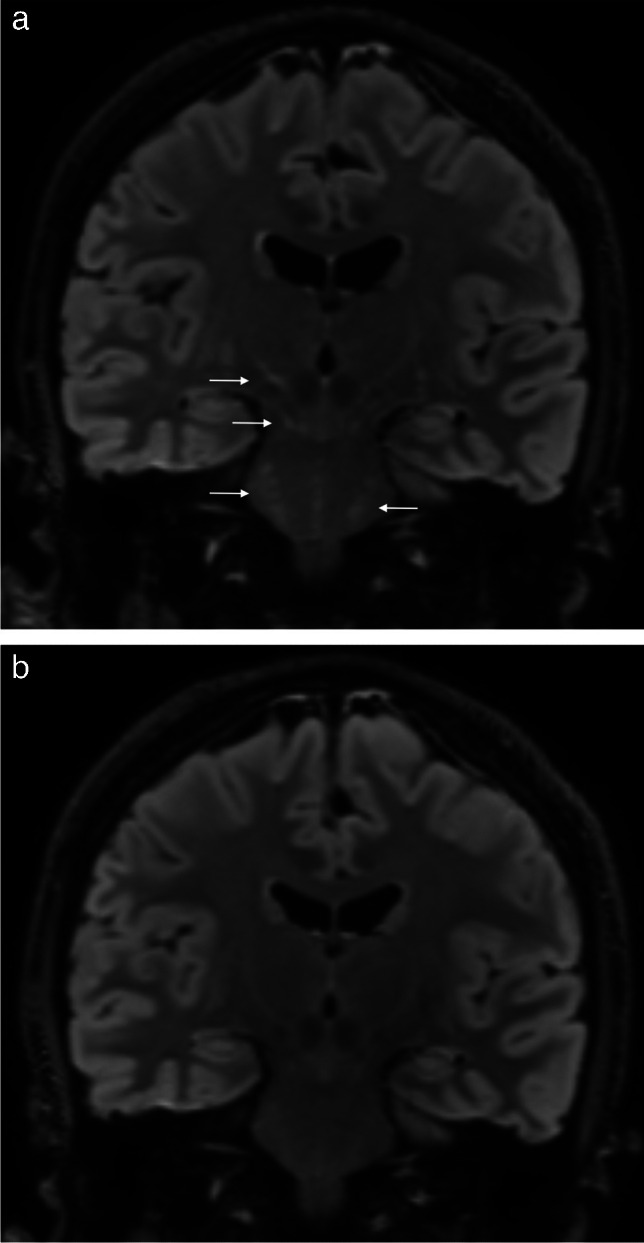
Encephalitis. Coronal FLAIR images from definite neuroborreliosis case with encephalitis showing small hyperintensities (arrows) in right crus cerebri, mesencephalon and pons bilaterally at baseline (a) (hyperintense changes were also seen in internal capsules and cerebellar dentate nuclei, not shown here). There was complete regression of the findings at follow-up (b)
